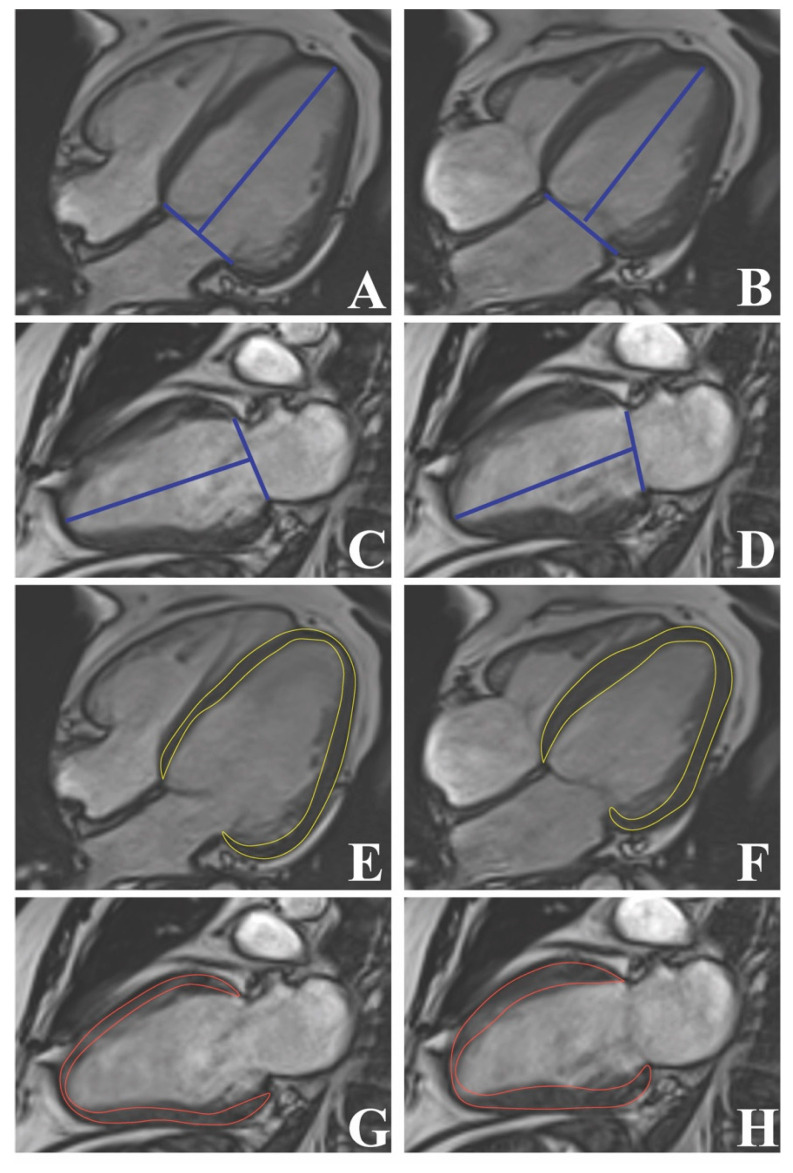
Left ventricular geometry and strain by cine-cardiac magnetic resonance imaging: Left ventricle long-axis strain (A–D) and sphericity index (E–H).
